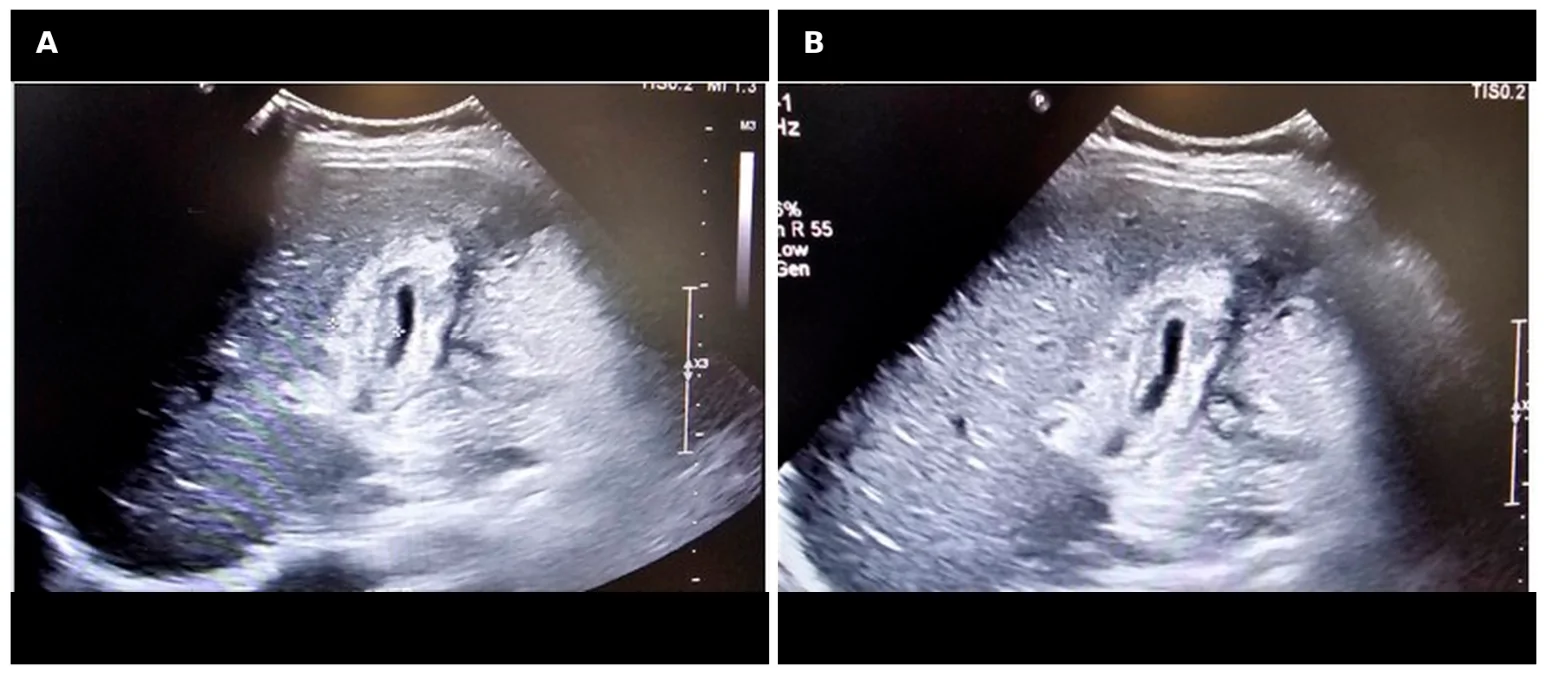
Abdominal ultrasonography of the gallbladder in two planes (A,B). The gallbladder is contracted with marked, diffuse wall thickening measured at 1.87 cm (callipers) and surrounding inflammatory change; no calculi are seen.
